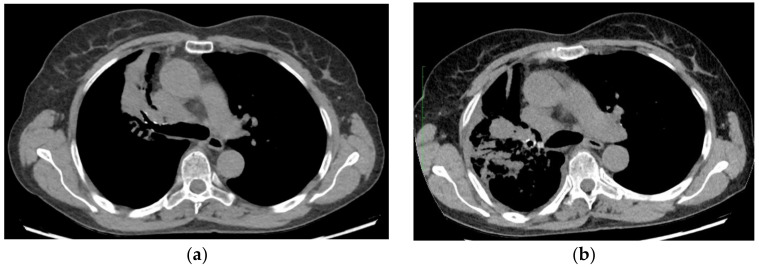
Computed tomography (CT) images of the lung (a) CT scan 1 month after first osimertinib administration (b) CT scan 3 months after first osimertinib administration.
